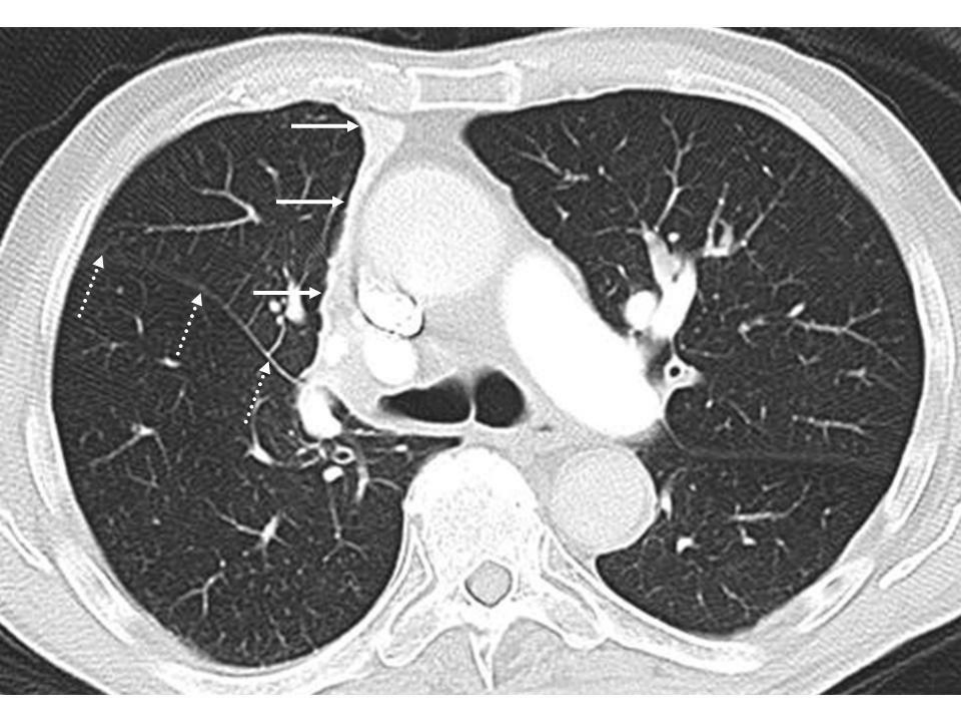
An axial CT image (lung window) of a 62 years old smoker with partial collapse of the right upper lobe secondary to a bronchogenic carcinoma shows anterior displacement of the right oblique fissure (dotted arrows). Solid arrows point to the lateral margin of the collapsed segment of the right upper lobe. Bronchogenic carcinoma is not seen on this image. Left oblique fissure is normal in its position
